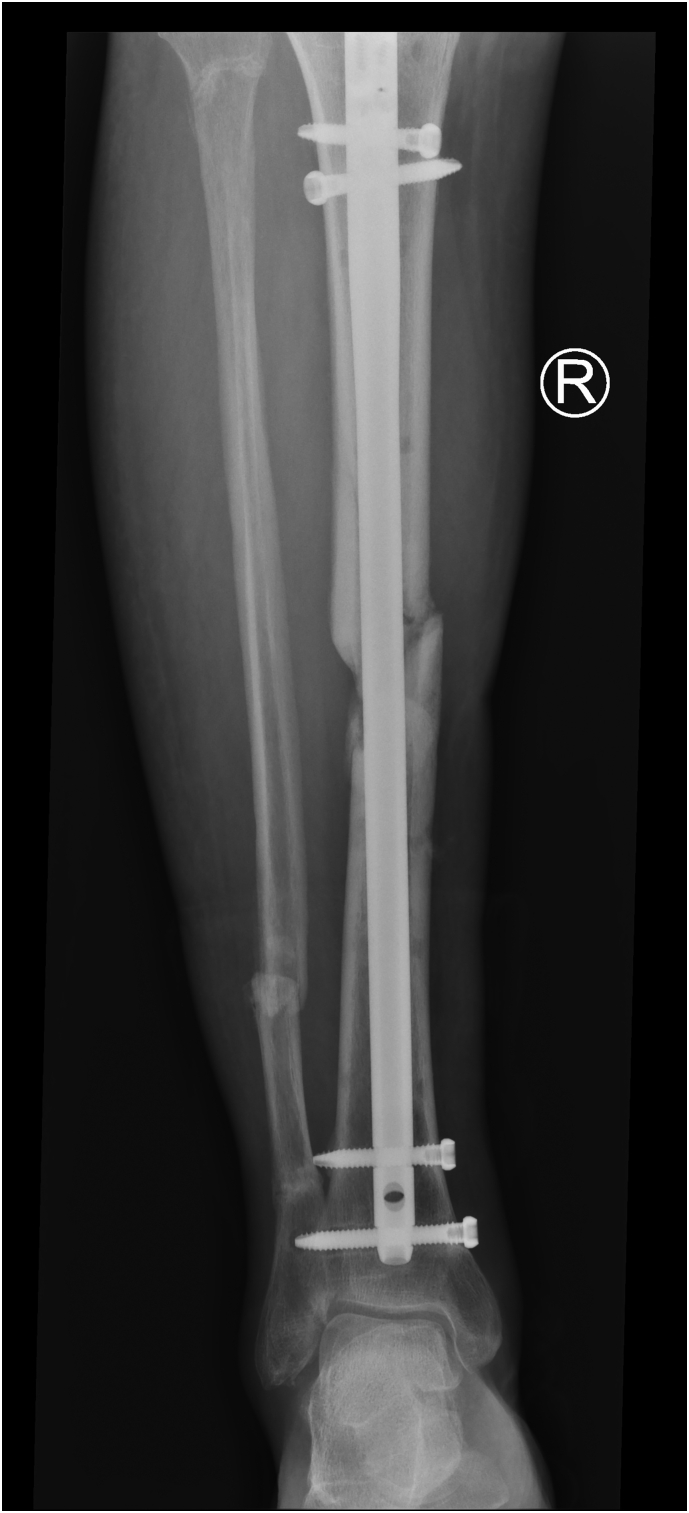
Anterior posterior radiograph of the right leg showing IM nail in situ.
